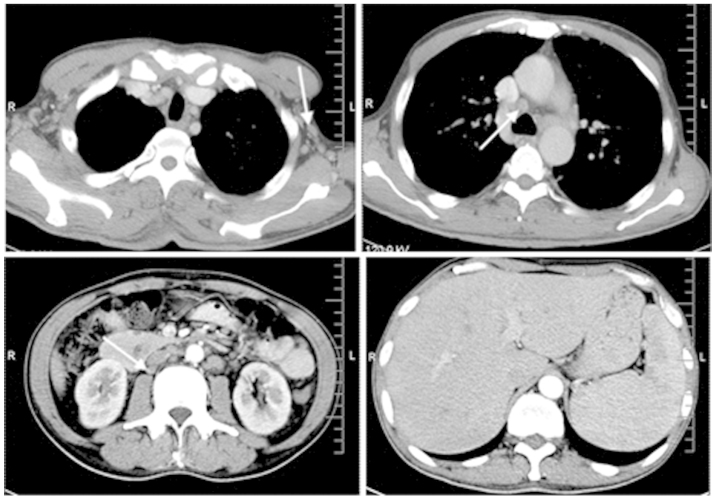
Computed tomography scans revealing swelling of the axillary (top left image), mediastinal (top right image) and retroperitoneal (bottom left image) lymph nodes, and hepatosplenomegaly (bottom right image). Arrows indicate the areas of swelling.
